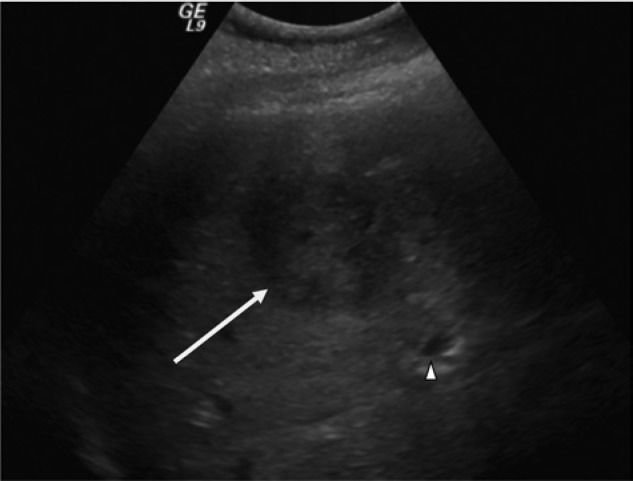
Right upper quadrant ultrasound. A space-occupying lesion (white arrow), consistent with a pyogenic liver abscess, is visible in the right lobe of the liver. Dilated intrahepatic ducts (white arrowhead) can also be seen.
